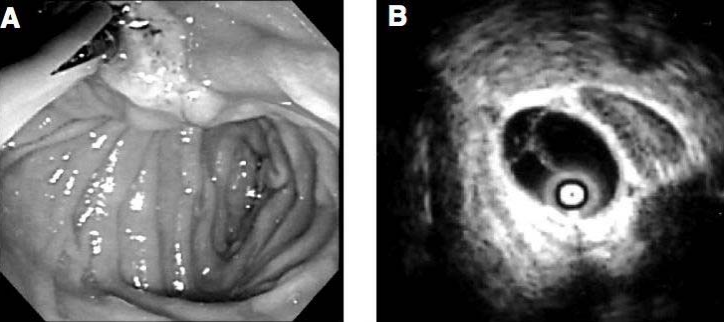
Endoscopic image of the duodenum shows mucin draining from a patulous papillary orifice (A). Endoscopic ultrasonography showed no mass protruding into the lumen in the bile duct and the cystic lesion at the left lateral segment of the liver (B).
